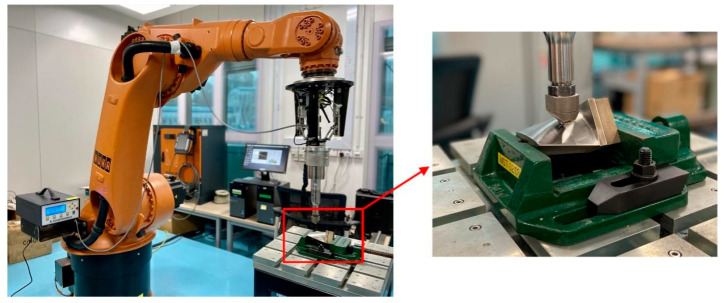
Robotic ultrasonic strengthening on aviation blade surface.
